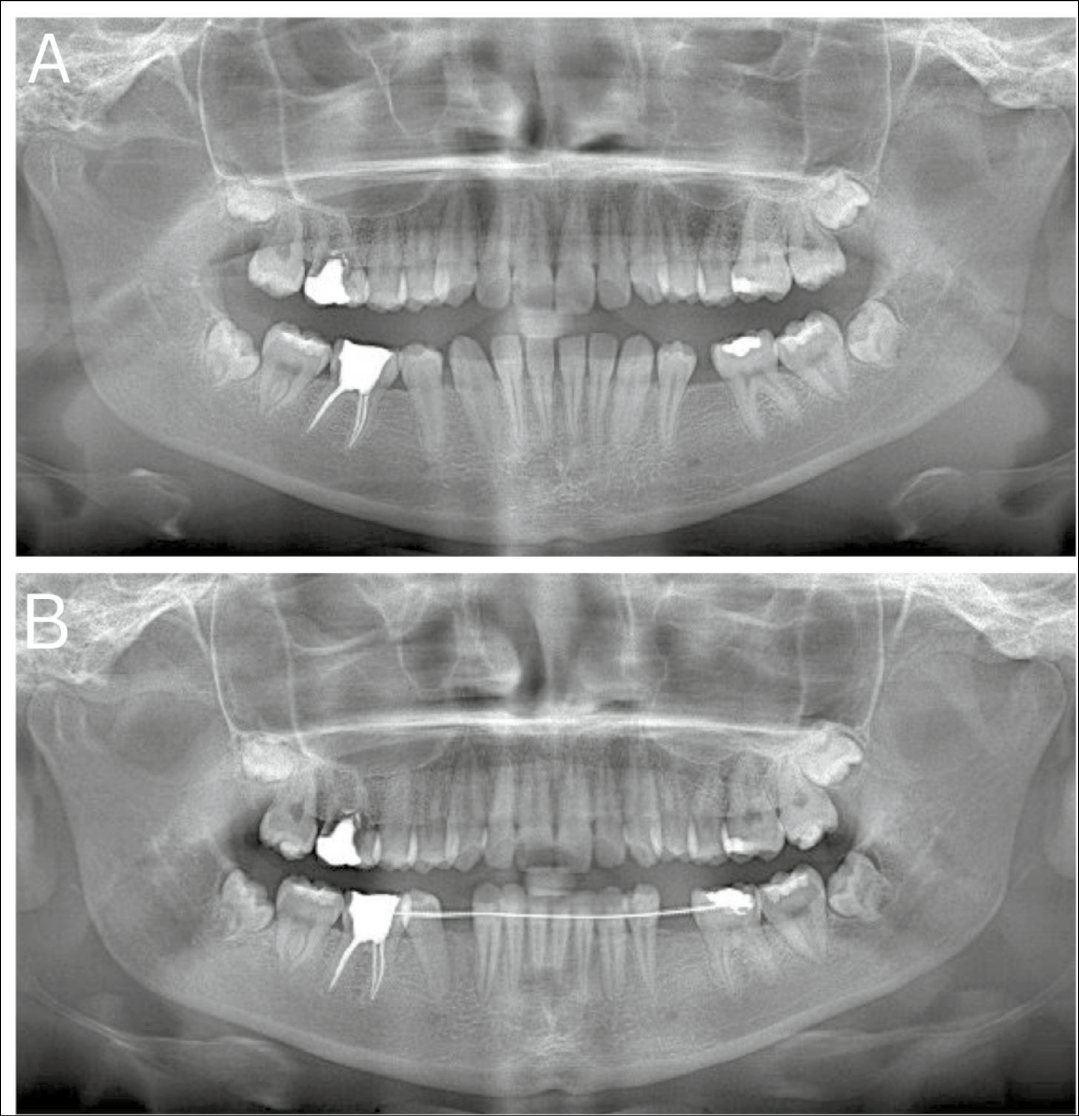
Orthopantomogram progression showing worsening root resorption of tooth 16 and delayed decision for autotransplantation. A (age 16 years) Panoramic radiograph taken 6 months after radiotherapy showing progressive root resorption of tooth 16. Initial consideration of autotransplantation was rejected at this stage. B (age 18 years) Follow-up panoramic radiograph taken 1 year later demonstrating further root resorption of tooth 16, with worsened structural integrity. Based on disease progression, autotransplantation was approved. Note the presence of orthodontic appliances initiating space preparation. Both images were taken before surgical intervention
